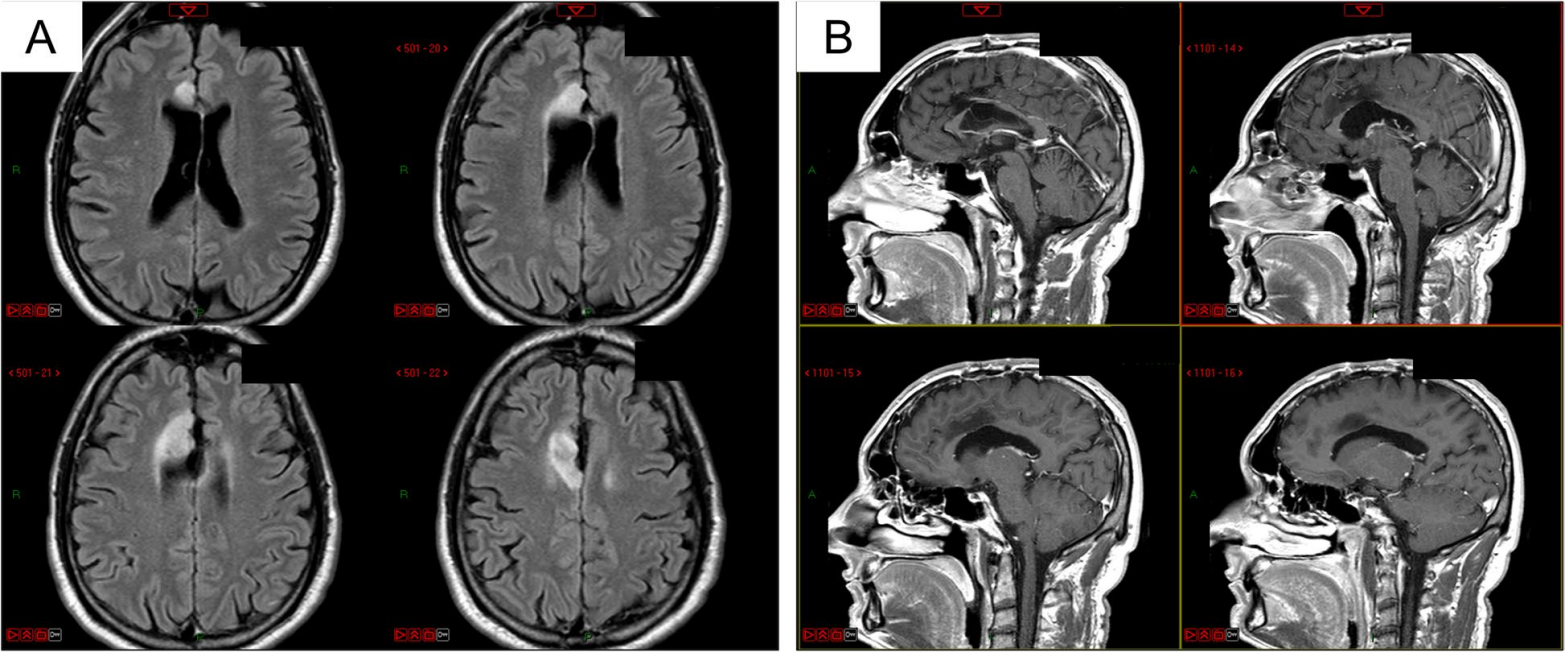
Preoperative FLAIR (A) and contrast enhanced T1-weighted (B) magnetic resonance images showing a glioma in the right cingulate gyrus
